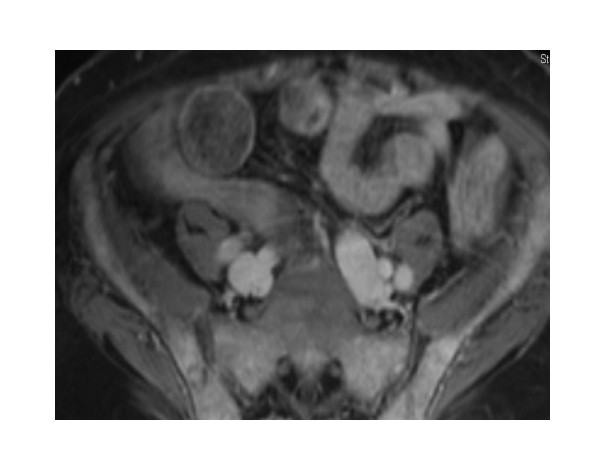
MRI pelvis. Diffuse bone marrow replacement throughout the pelvis and proximal femora with only small areas of residual fatty marrow in the greater trochanters and femoral heads bilaterally. The diffuse enhancement is consistent with extensive disease.
